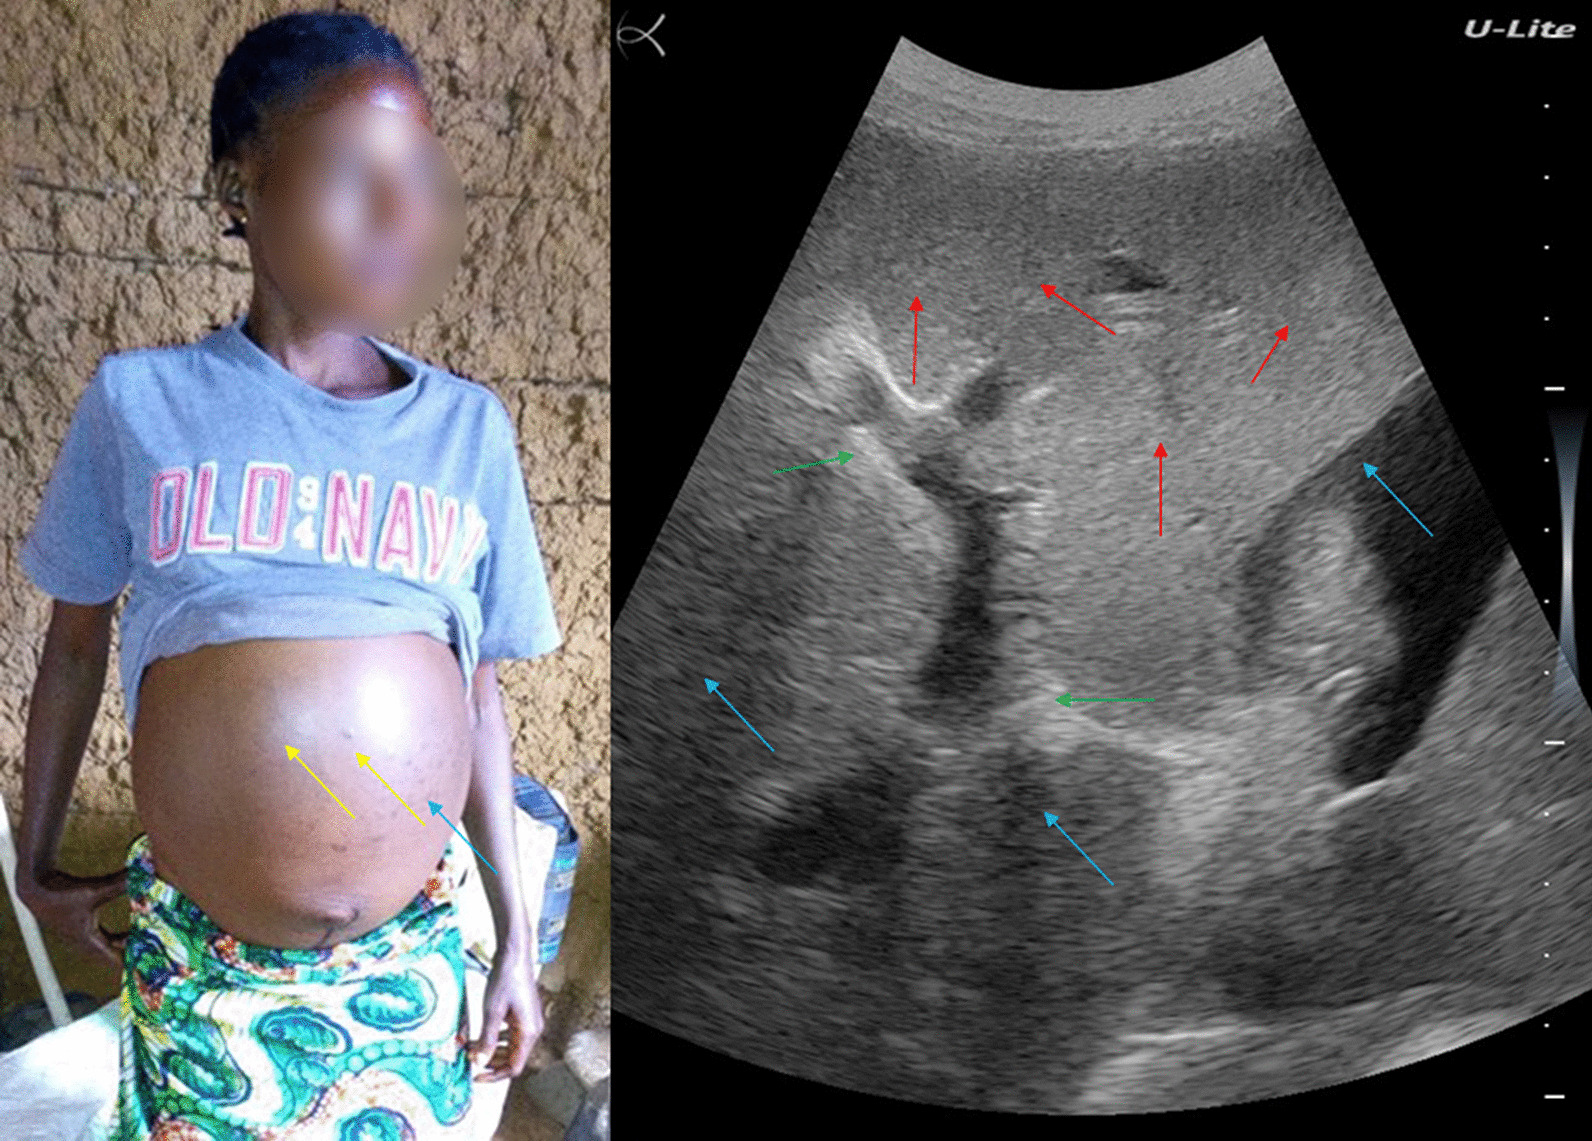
Patient #8, a young woman with a big belly, collaterals and abdominal rash spots (yellow arrows, left). Ultrasound examination (right) showing liver with distorted architecture, nodular surface, heterogeneous and starry night sky echotexture of the parenchyma (red arrows), decreased definition of the portal vein wall (green arrow), and ascites (blue arrow)
